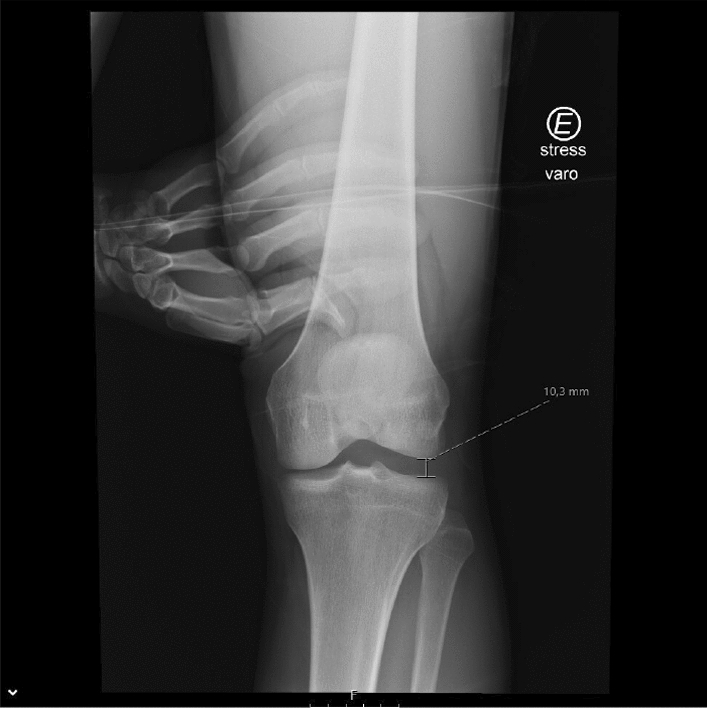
X-ray image with hands of a physician whilst performing the varus stress on the left knee (“E” is an indicator for “Left”).
